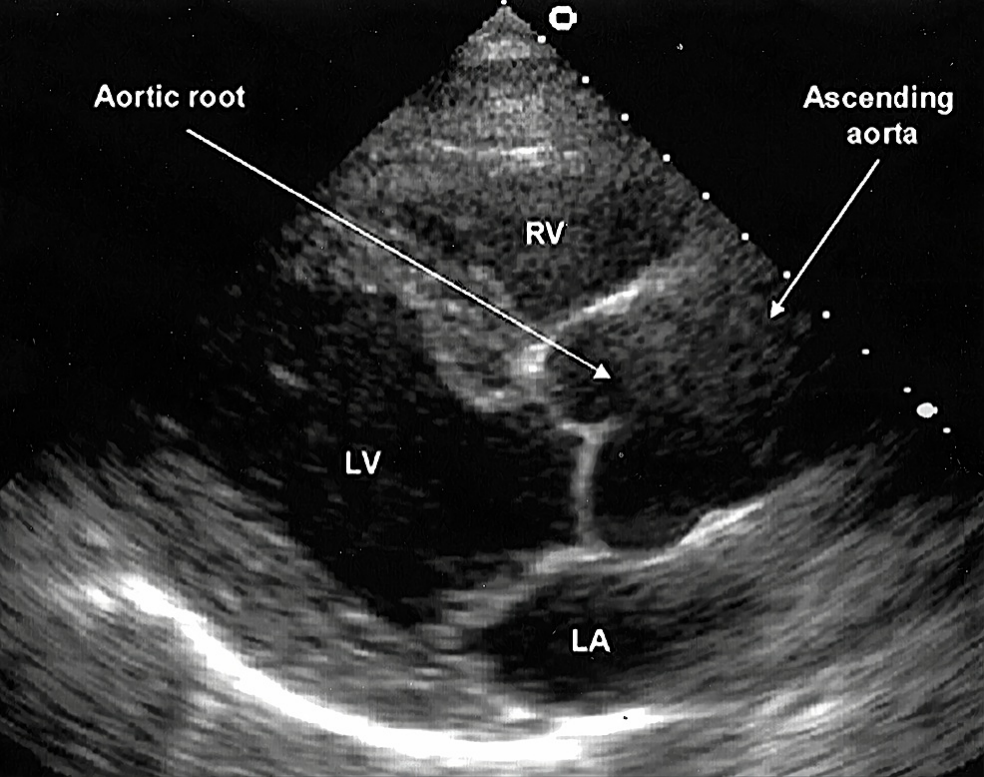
Transthoracic echocardiogram showing normal findings
